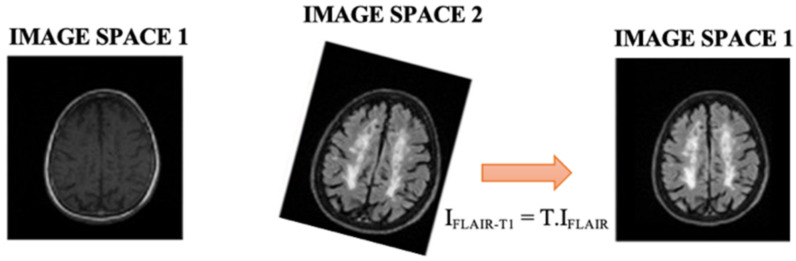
FLAIR volume spatially aligned to T1-w volume.
